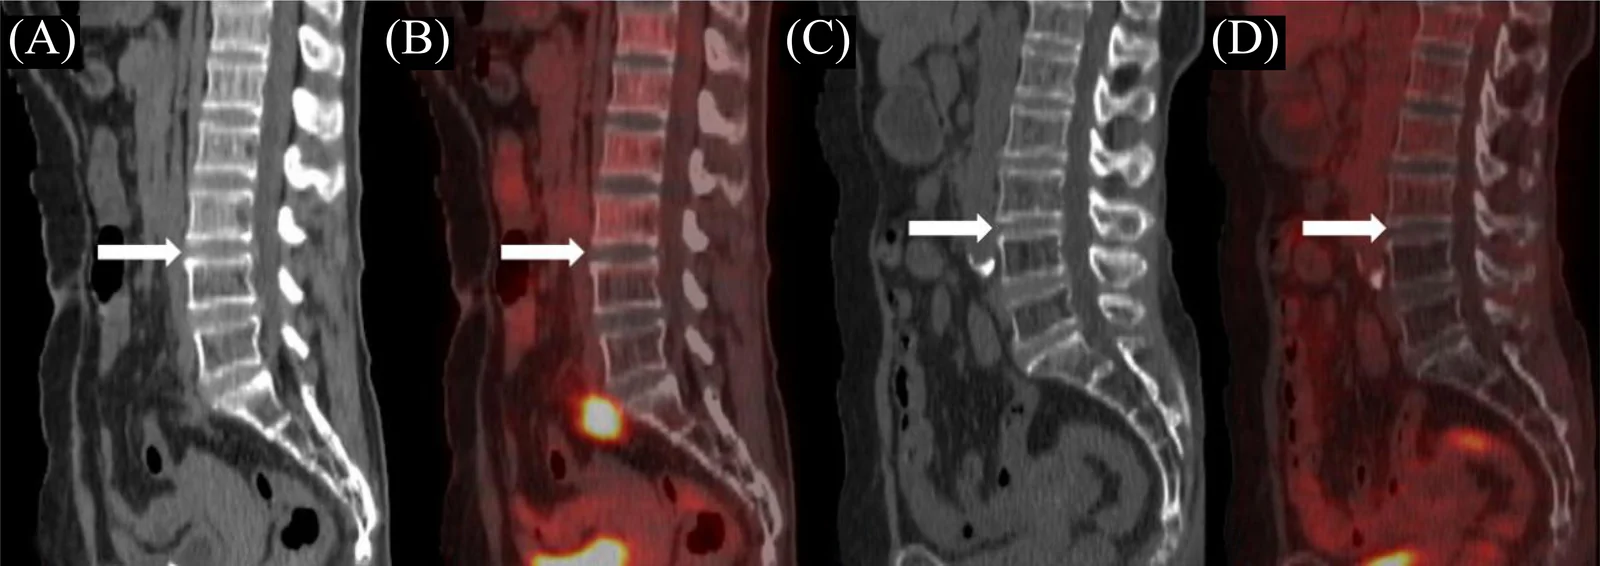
Sagittal CT and PET/CT fusion images demonstrate radiation-induced Sacral Insufficiency Fractures (SIFs) in two cervical cancer patients post-radiotherapy. In the 52-year-old female (7 months post-RT), CT reveals markedly reduced vertebral density within the irradiated field (39 HU) compared to non-irradiated bone (110 HU) at the L3-L4 boundary (arrow ↑, A), with correspondingly lower FDG uptake (SUVmax 0.56 *vs.* 1.65; arrow, B). Similarly, the 65-year-old female (13 months post-RT) shows more pronounced osteopenia in irradiated vertebrae (5 HU *vs.* 59 HU; arrow, C) and suppressed metabolic activity (SUVmax 0.70 *vs.* 2.01; arrow, D), both delineated by the L3-L4 interface.
